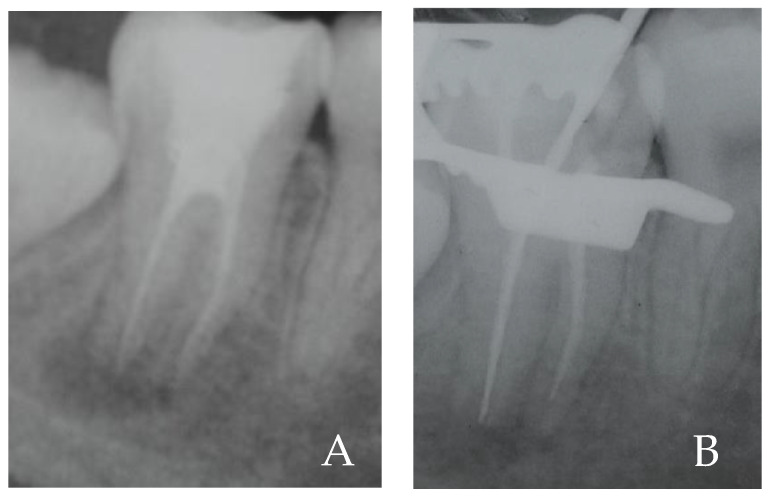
(A) Dental element 47 with radiolucent image in the periapical region. (B) Retreatment of inferior second right molar, with periapical lesion, after working length confirmed by intraoperative X-ray.
